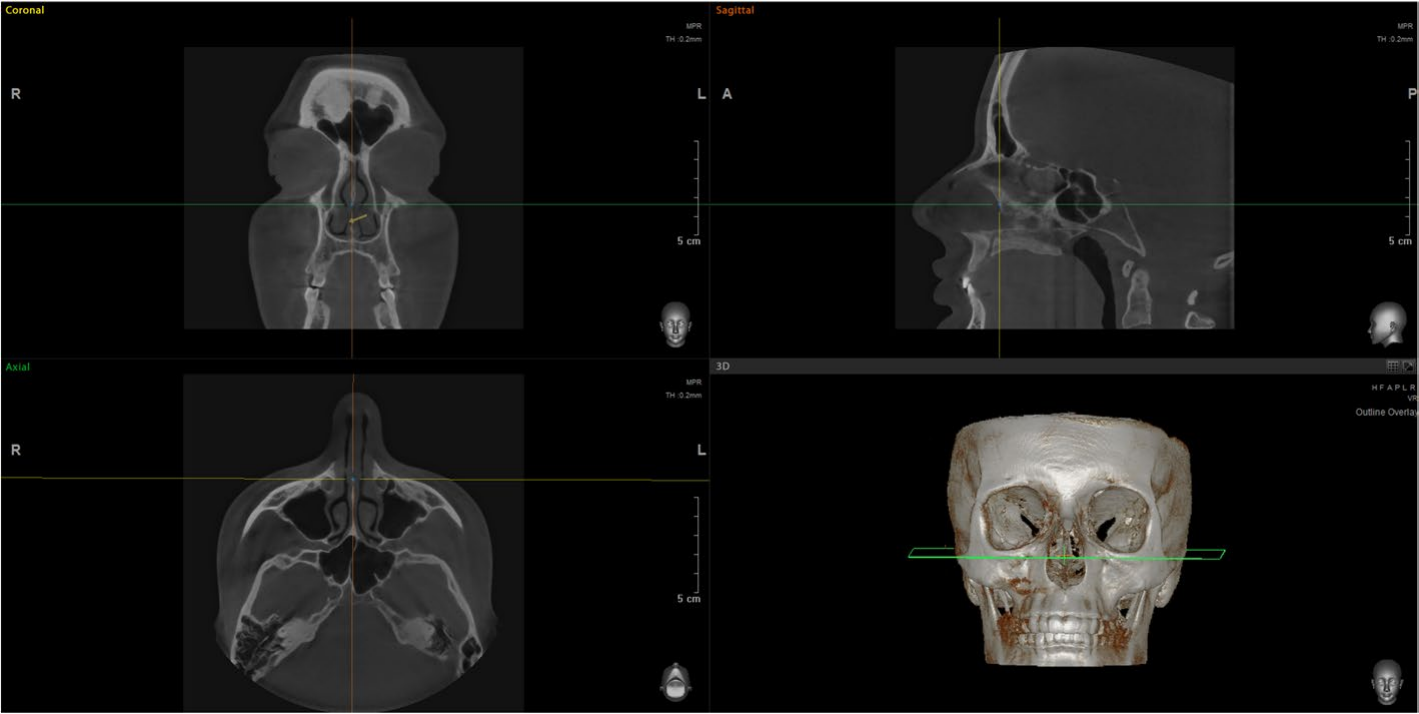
Computed tomography of the nasal cavity prior to CT-assisted precision rhinoplasty, depicting the distortions of the narrow internal nasal valves, hypertrophic turbinates, soft tissues thickening of sidewalls and septum, dimensions and relationships between chondral septum, ethmoid bone, and vomer; 3D reconstruction is extremely useful for precise understanding of anatomy for individualized surgical plan and predictable maneuvers focused on specific nuances
